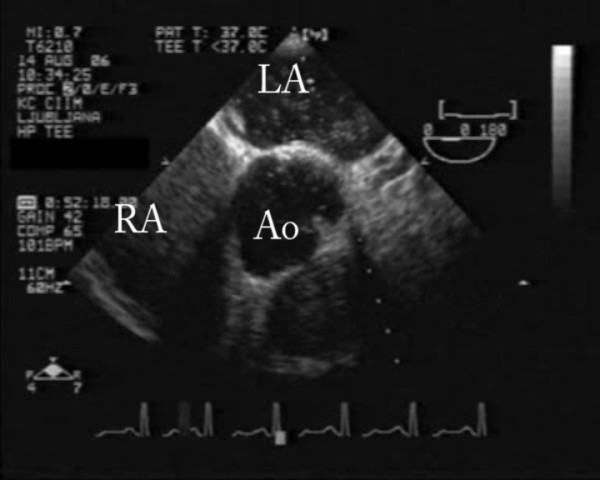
Transesophageal echocardiography showing right to left intra-atrial shunt after contrast application. Ao- aorta, LA- left atria, RA- right atria
